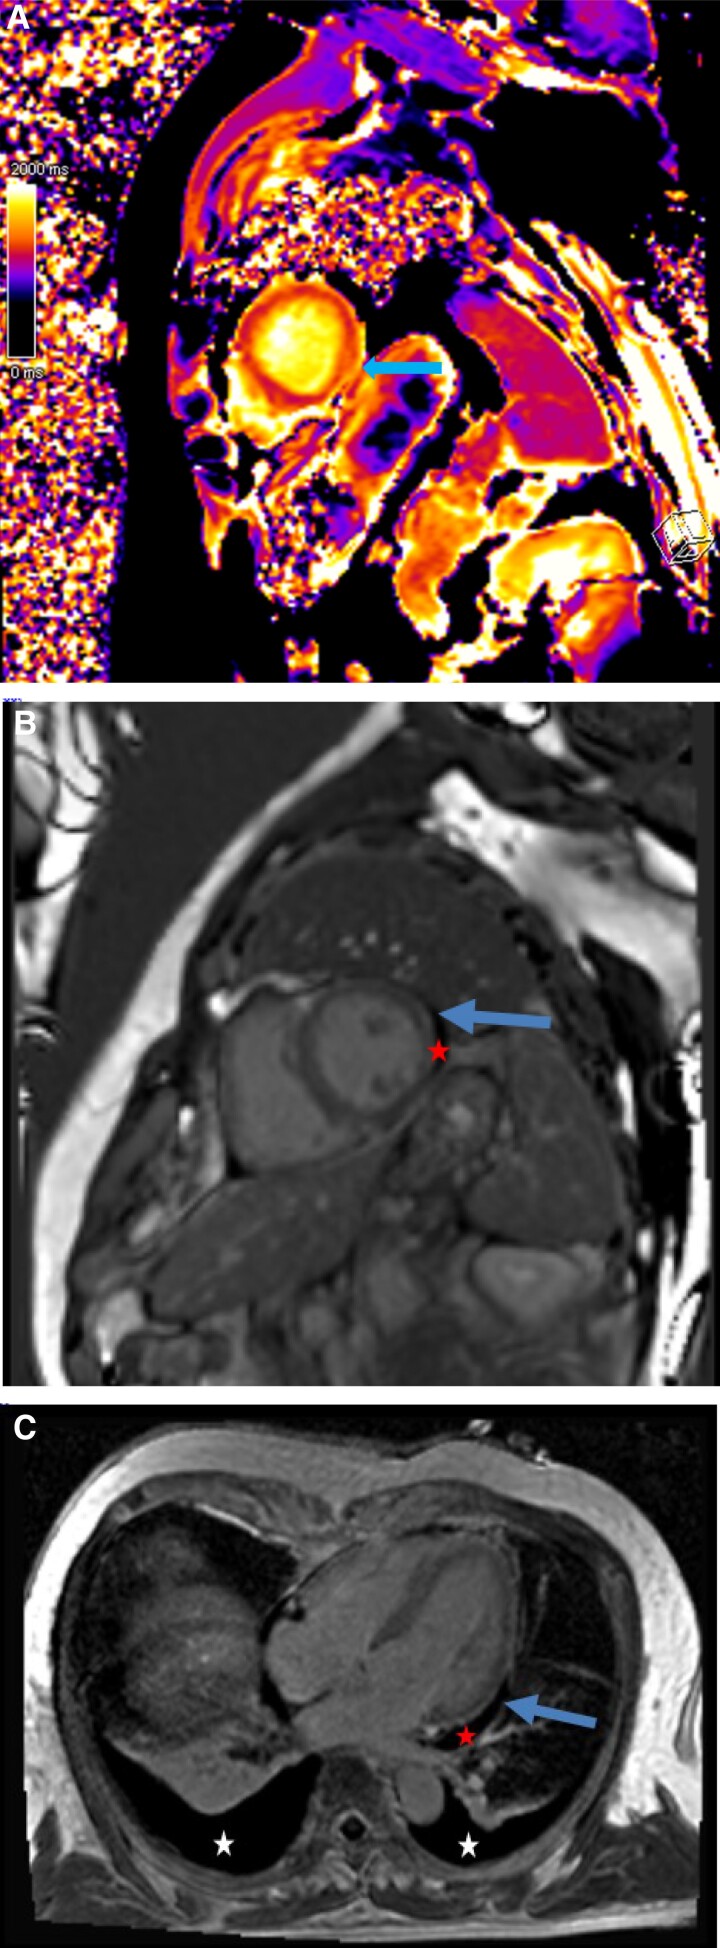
Cardiac MRI displaying subepicardial gadolinium chelate enhancement particularly in the anterolateral and basal inferolateral segment (blue arrow) with pericardial effusion (red star) in late phase-sensitive inversion recovery (PSIR) acquisition in short-axis (*B*) and apical four-chamber long-axis (*C*) views. Native T1 mapping sequence displays an increase in T1 relaxation time up to 1366 ms in the subepicardial area in the same segment (*A*, blue arrow), whereas T1 relaxation time is evaluated at 1200 ms in safe myocardium (T1 value of normal myocardium is 950–1000 ms). Acute myocyte lesions are corroborated by mapping, but the extension of fibrosis cannot be precisely evaluated considering the oedematous component. Note the bilateral pleural effusion (white star) in the apical four-chamber long-axis (*C*) view in this patient with lightly altered cardiac function (left ventricular ejection fraction = 40%). No atrial dilation or intraventricular thrombus is found.
